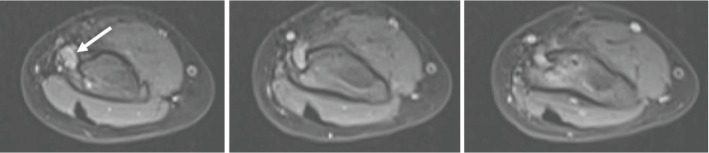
T2 DPFS MRI sequence showing hypertrophy (white arrow) and median nerve entrapment within the consolidated fracture.
